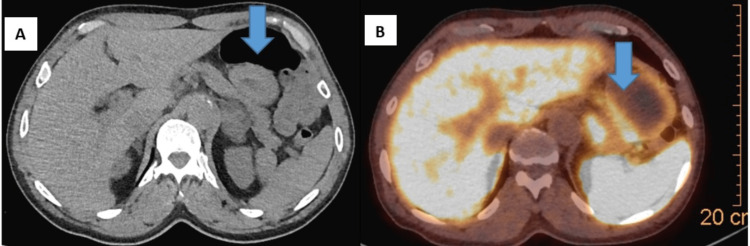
(A) CT scan of the abdomen and pelvis with and without contrast. A 4.1 x 3.1 cm left adrenal mass (arrow) with focus of macroscopic fat posteriorly highly supportive of adrenal myolipoma. (B) On GA-68 DOTATATE in the medial inferior aspect, there is relatively focal, moderate to intense radiotracer activity noted (Krenning score 3).
